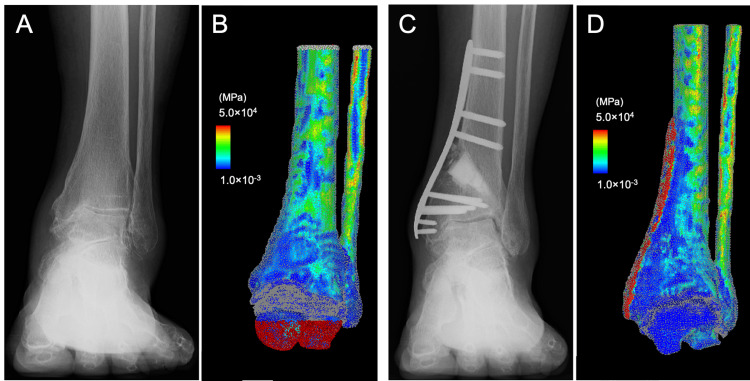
Case 2: A 65-year-old woman with stage 3a osteoarthritis of the left ankle. (A) preoperative anteroposterior weight-bearing radiograph of the ankle (B) the corresponding finite-element model with distribution of the elastic modulus (C) postoperative anteroposterior weight-bearing radiograph of the ankle, and (D) the corresponding finite-element model with the distribution of the elastic modulus at six months after distal tibial oblique osteotomy
